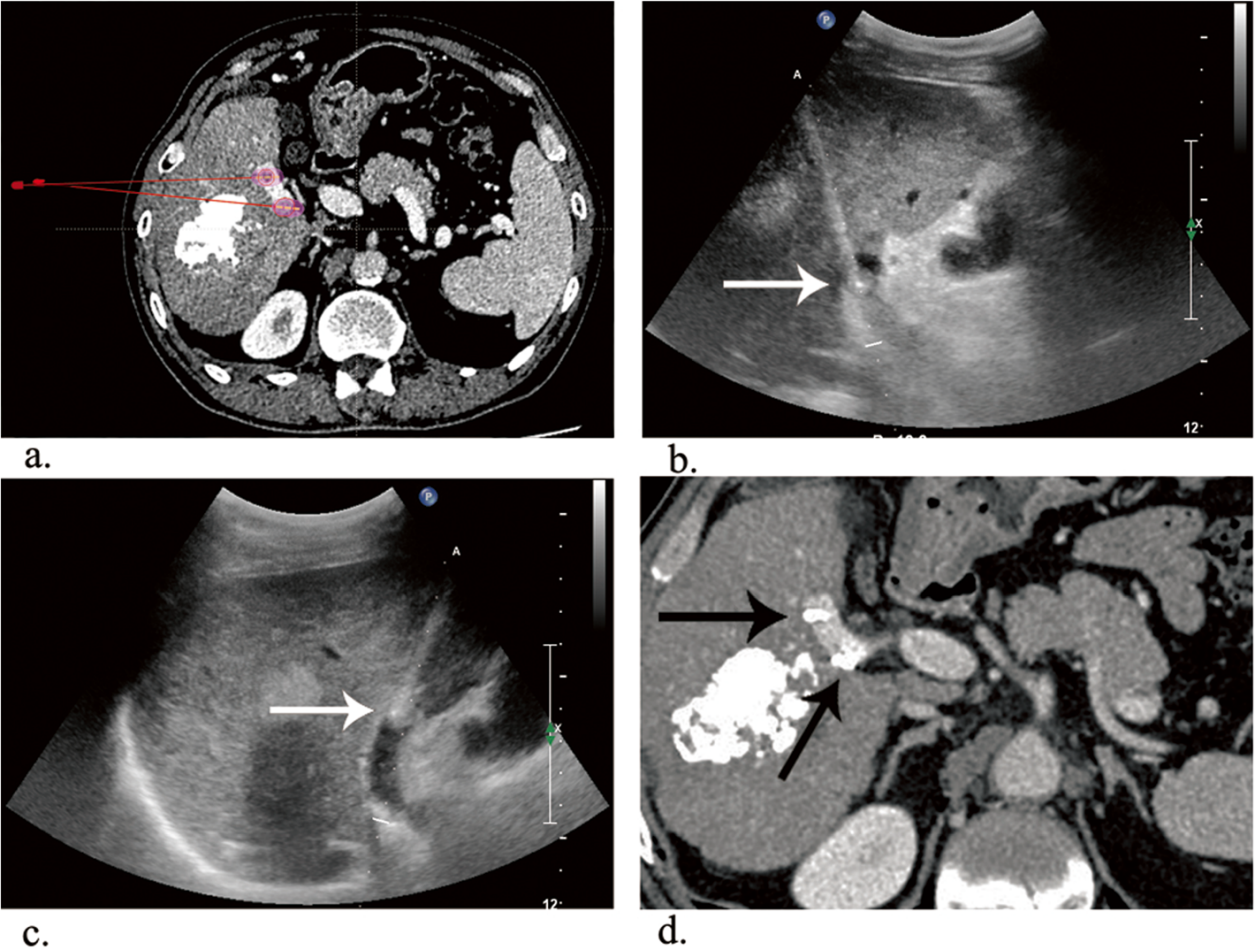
a Contrast-enhanced CT of two 1-cm diameter tumor thrombi floating in the right segment of the portal vein. The preprocedural Treatment Planning System (TPS) was applied. b The needle (white arrow) punctured the proximal thrombus with ultrasound guidance. c The needle (white arrow) was placed in the distal thrombus. d Contrast-enhanced CT at the 1-month follow-up. Tumor response was evaluated as CR (black arrows)
